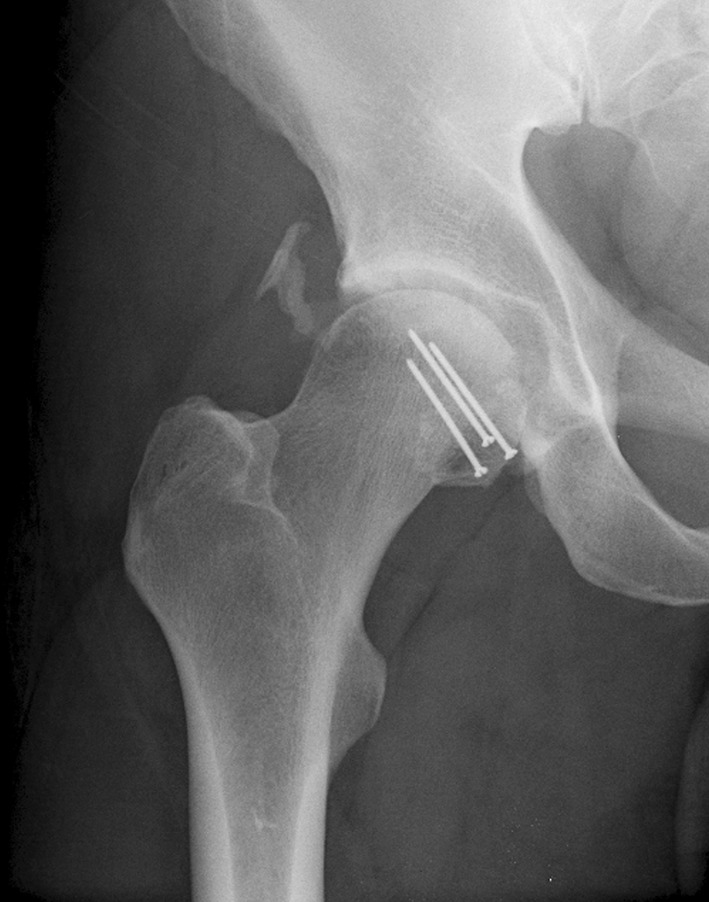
AP radiograph of a 37-year-old patient 15 months after ORIF of a femoral head fracture with non-bridging Brooker I heterotopic ossification
